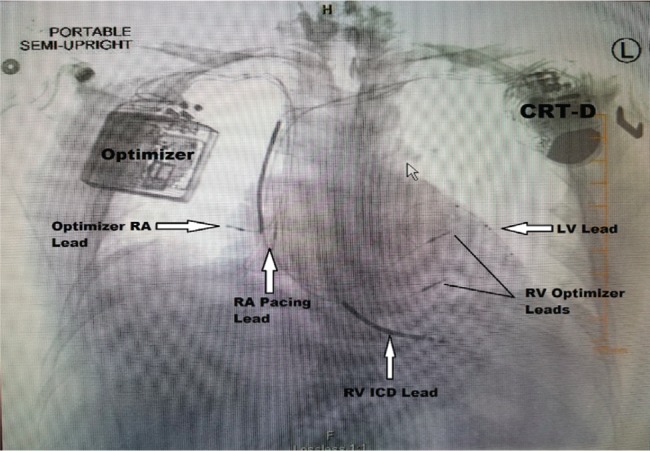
Postprocedure anteroposterior chest radiograph, revealing no change occurred in lead position.
